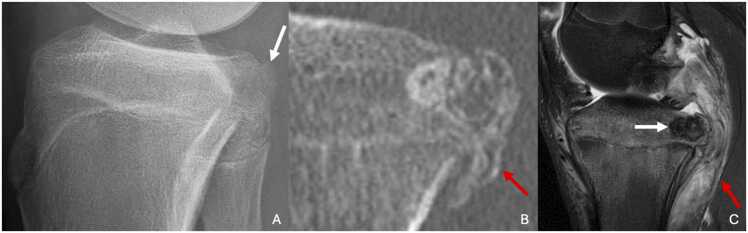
Chondroblastoma in 15-year-old male adolescent with post-traumatic right gonalgia. A. Profile radiography showing a radiolucency on the posterior part of the tibial plateau (white arrow). B. CT image in the sagittal plane highlighting an osteolytic lesion with sclerotic margins of the proximal epiphysis of tibia associated with periosteal reaction (red arrow). C. T2-weighted fat-suppressed MR image in the sagittal plane showing a pronounced hyposignal of the lesion, characteristic of chondroblastoma (white arrow), surrounding by bone marrow edema and intense adjacent soft-tissue inflammatory reaction (red arrow).
